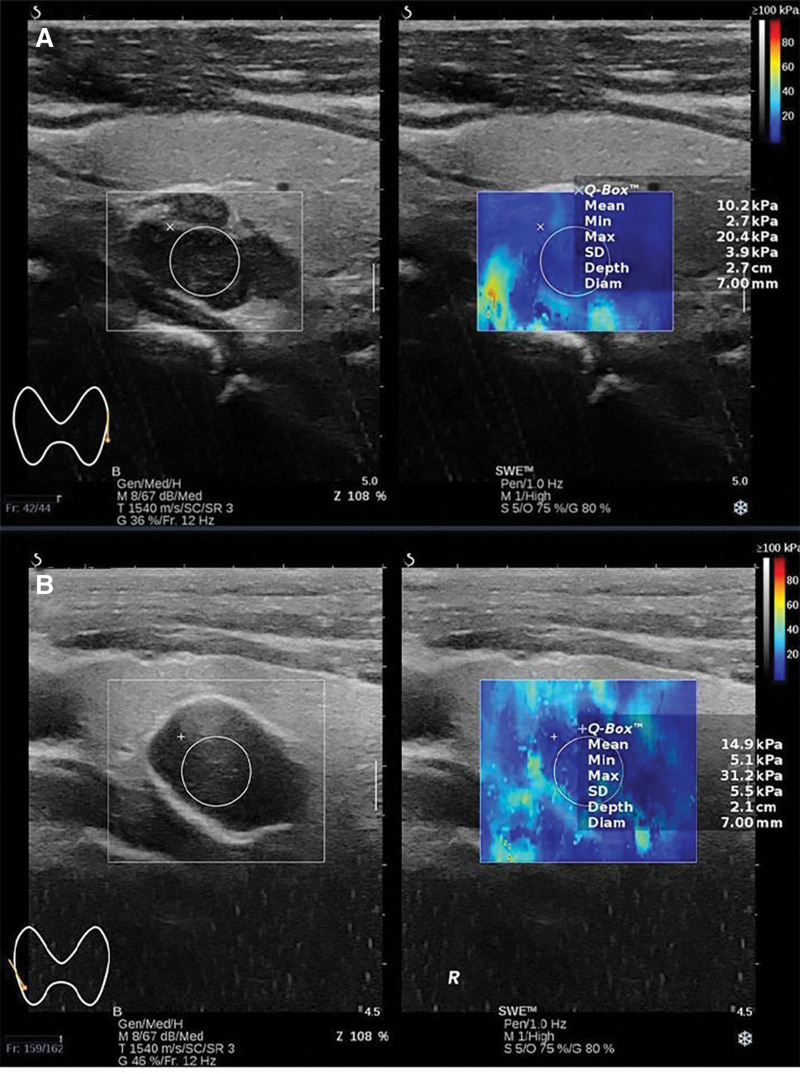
(A) Shear-wave ultrasound imaging of secondary hyperparathyroidism. (B) Shear-wave ultrasound imaging of secondary parathyroid hyperplasia. It is obvious that the Young’s modulus value of secondary parathyroid carcinoma is significantly higher than that of secondary hyperparathyroidism.
